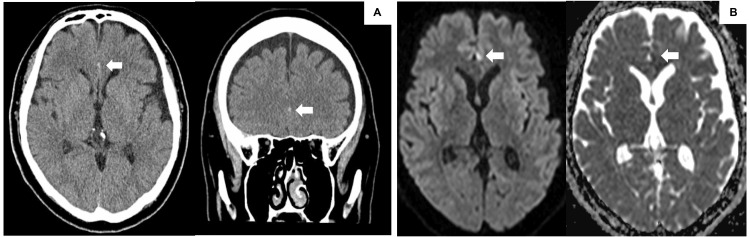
Images at onset of our patient at admission to our department. (A) Computed tomography scan on admission reveals subarachnoid hemorrhage in the interhemispheric fissure (arrows). (B) Magnetic resonance imaging (diffusion-weighted image and apparent diffusion coefficient map image) on admission revealing acute cerebral infarction in the left cingulate gyrus (arrows).
